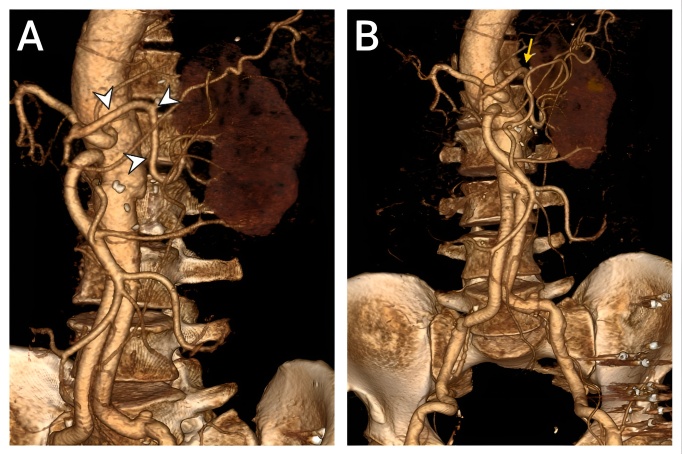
A) Postoperative 3D CT reconstruction in the left anterior oblique view demonstrates a patent splenorenal bypass (arrowheads). B) Anterior view shows the bypass (arrow) along with the reconstructed abdominal aorta.
